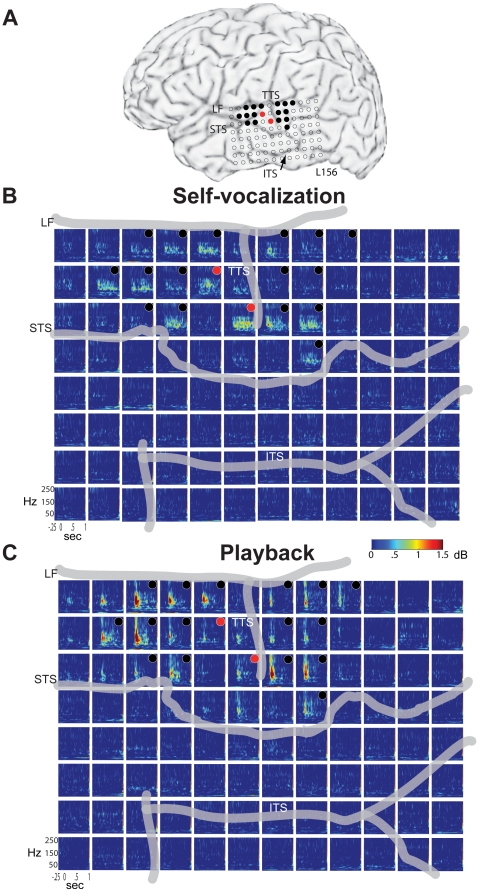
Time-frequency analysis of subject 156's responses during self-vocalization and playback. (A) MRI surface rendering of the left cerebral hemisphere showing the locations of all recording contacts and major sulci. In this figure, filled black circles denote contacts where significant *decreases* (p<.01, 0–500 msec post-stimulus) in high gamma band (HGB, 70–150 Hz) power occurred during self-vocalization (SV) compared to playback (PB). Red circles indicate contacts where significant *increases* in high gamma band power were observed during SV compared to PB. (B) Broad-band time-frequency analysis (2–250 Hz) of evoked responses recorded during SV. Individual panels display the power responses for each frequency band at each recording site (−.25 sec to 1 sec post-voice onset). The largest responses are seen to occur between 70–150 Hz. Thick gray lines represent the major sulci as labeled in A. (C) Time-frequency analysis of evoked responses recorded during the PB condition (LF-lateral fissure, STS-superior temporal sulcus, ITS-inferior temporal sulcus, TTS- transverse temporal sulcus).
